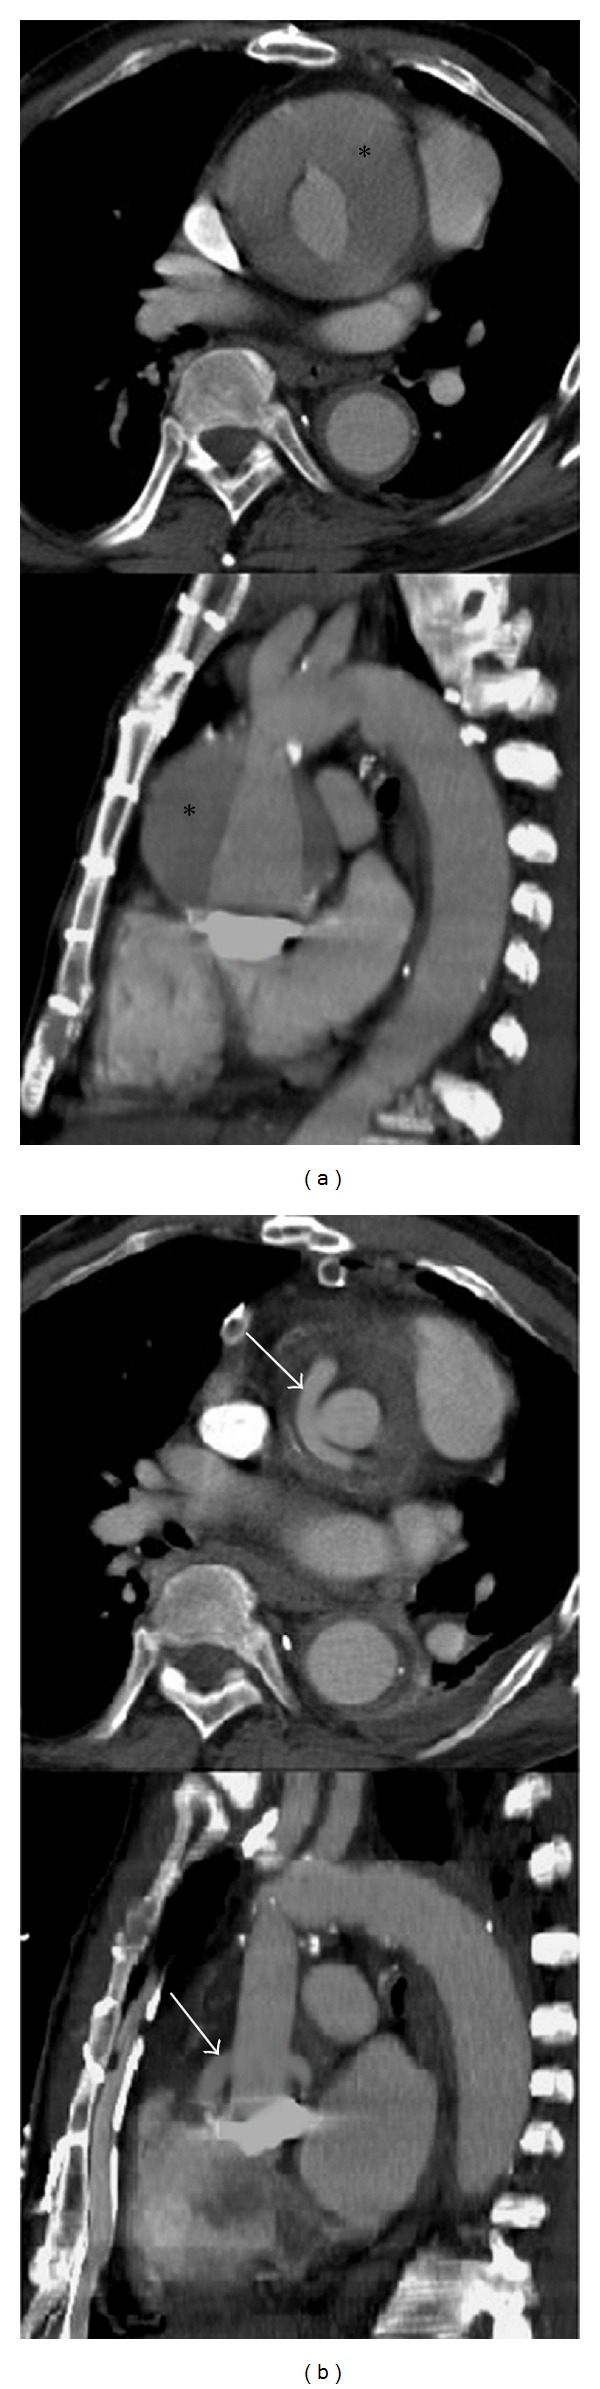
((a): top and bottom) Preoperative contrast enhanced CT scan showing the ascending aortic prosthesis, the mechanical aortic valve, and the surrounding hemorrhage within the former aneurysmal sac (black asterisk). ((b): top and bottom) Postoperative contrast enhanced CT scan showing the Cabrol interposition graft (white arrows).
